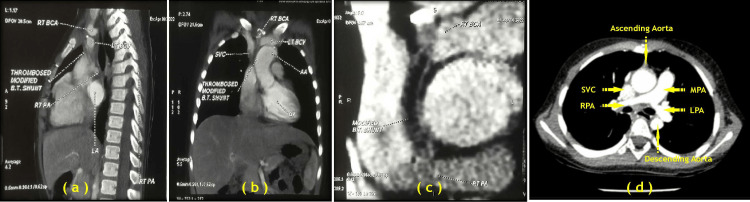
(a) CT sagittal reformation of blocked 4-mm sized PTFE-modified Blalock-Taussig shunt performed in a 5-year-old female child with tetralogy of Fallot with pulmonary stenosis with MAPCAs in October 2018; (b) CT coronal reformation of blocked modified Blalock-Taussig shunt; (c) CT curved oblique reformation of blocked modified Blalock-Taussig shunt; (d) Contrast CT axial image at the level of pulmonary arteries. BCA, Brachio-cephalic artery; BCV, Brachio-cephalic vein; AA, ascending aorta; SVC, superior vena cava; LA, left atrium; LV, left ventricle; PTFE, polytetrafluoroethylene; MAPCAs, major aorto-pulmonary collateral arteries; MPA, main pulmonary artery; RPA, right pulmonary artery; LPA, left pulmonary artery Image Credits: Dr. Deepakkumar V. Mehta
